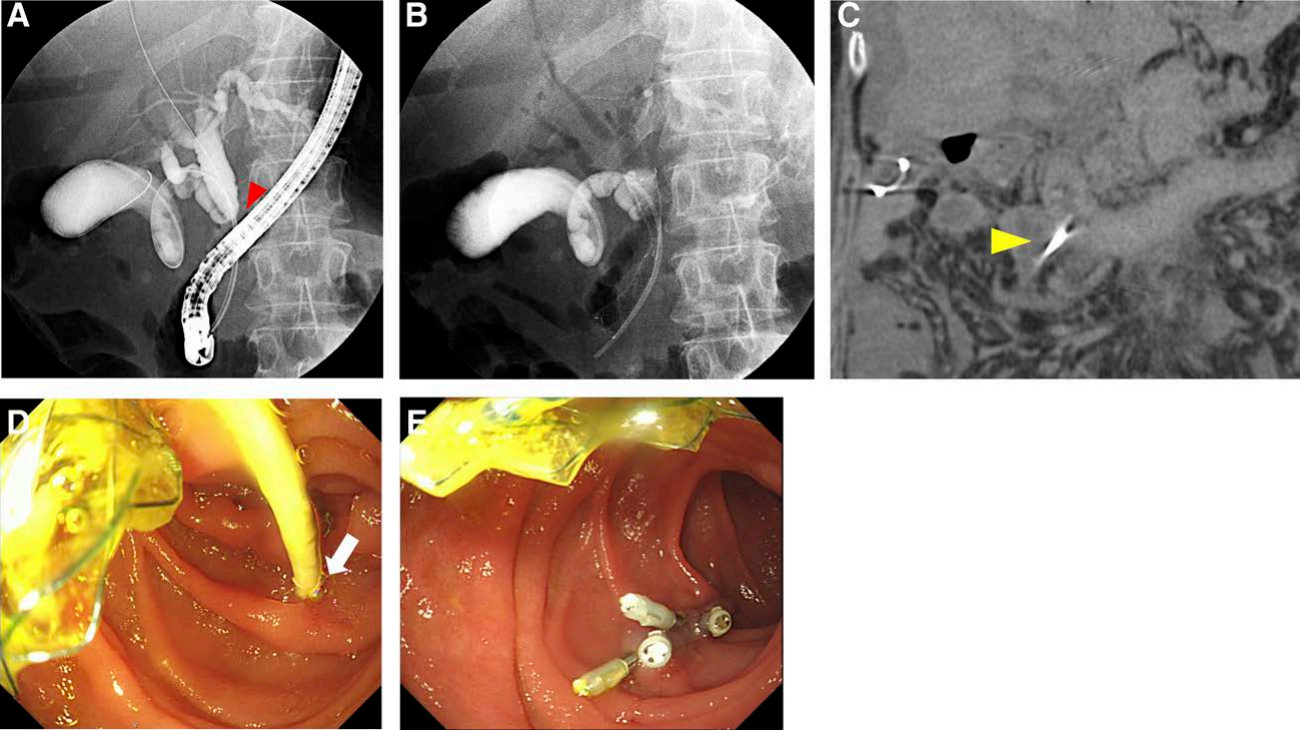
(A): ERCP revealed obstruction of the union of the cystic duct and the common bile duct (red arrowhead). (B): A 10 mm × 6 cm fully covered self-expandable metallic stent in the bile duct and an ENBD tube cut to 28 cm in the gallbladder to prevent cholecystitis were placed. (C): Abdominal CT revealed that the distal end of the gallbladder PS was protruding into the posterior peritoneum (yellow arrowhead). (D): The distal tip of the gallbladder stent penetrated the duodenal wall, which was confirmed during endoscopy (white arrow). (E): The perforation was closed using hemoclips. CT = computed tomography, ENBD = endoscopic nasal biliary drainage, ERCP = endoscopic retrograde cholangiopancreatography, PS = plastic stent.
